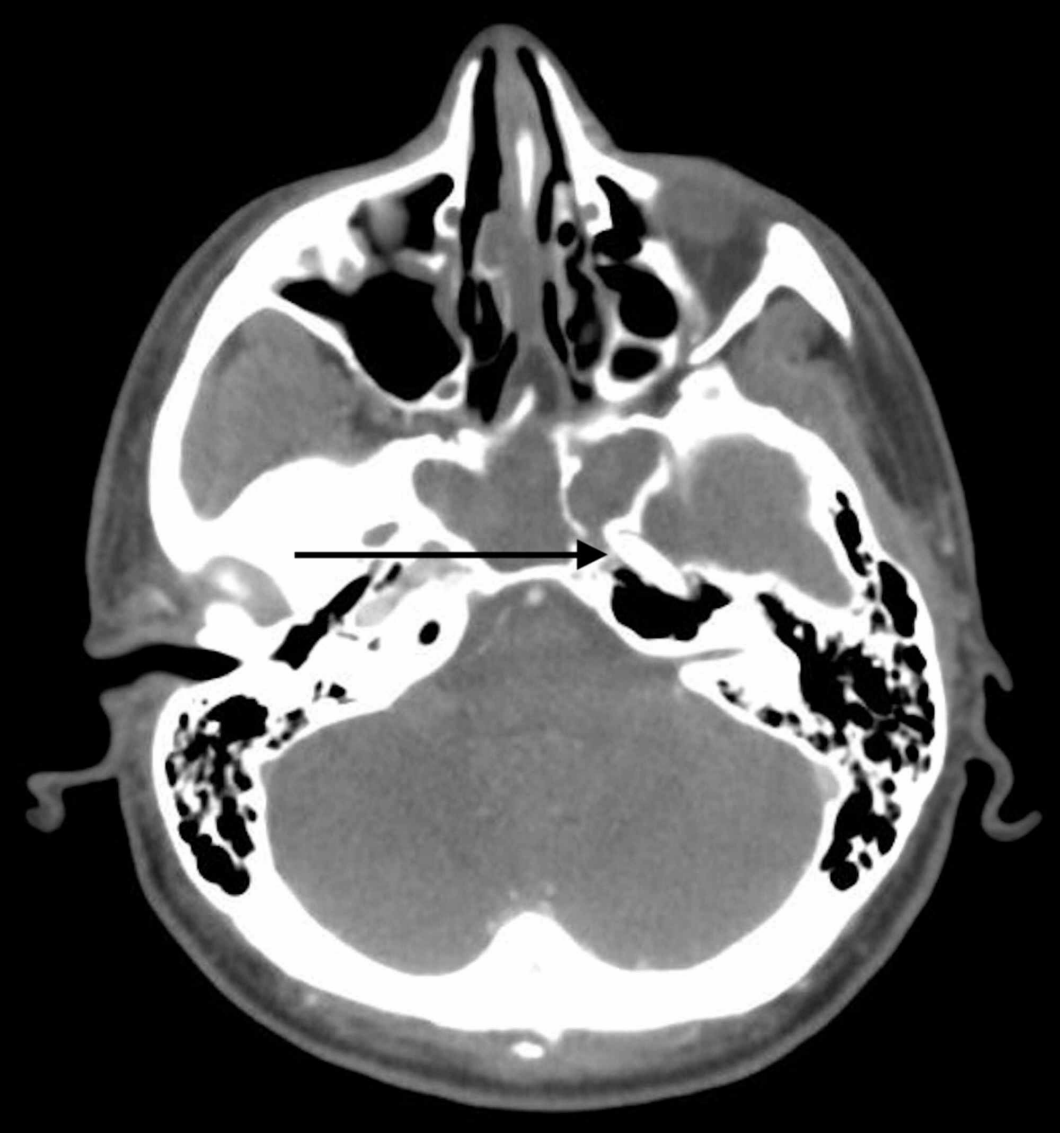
Follow-up CTA one week later The image shows the resolution of the left petrous carotid artery pseudoaneurysm (black arrow) CTA: computed tomography angiogram
